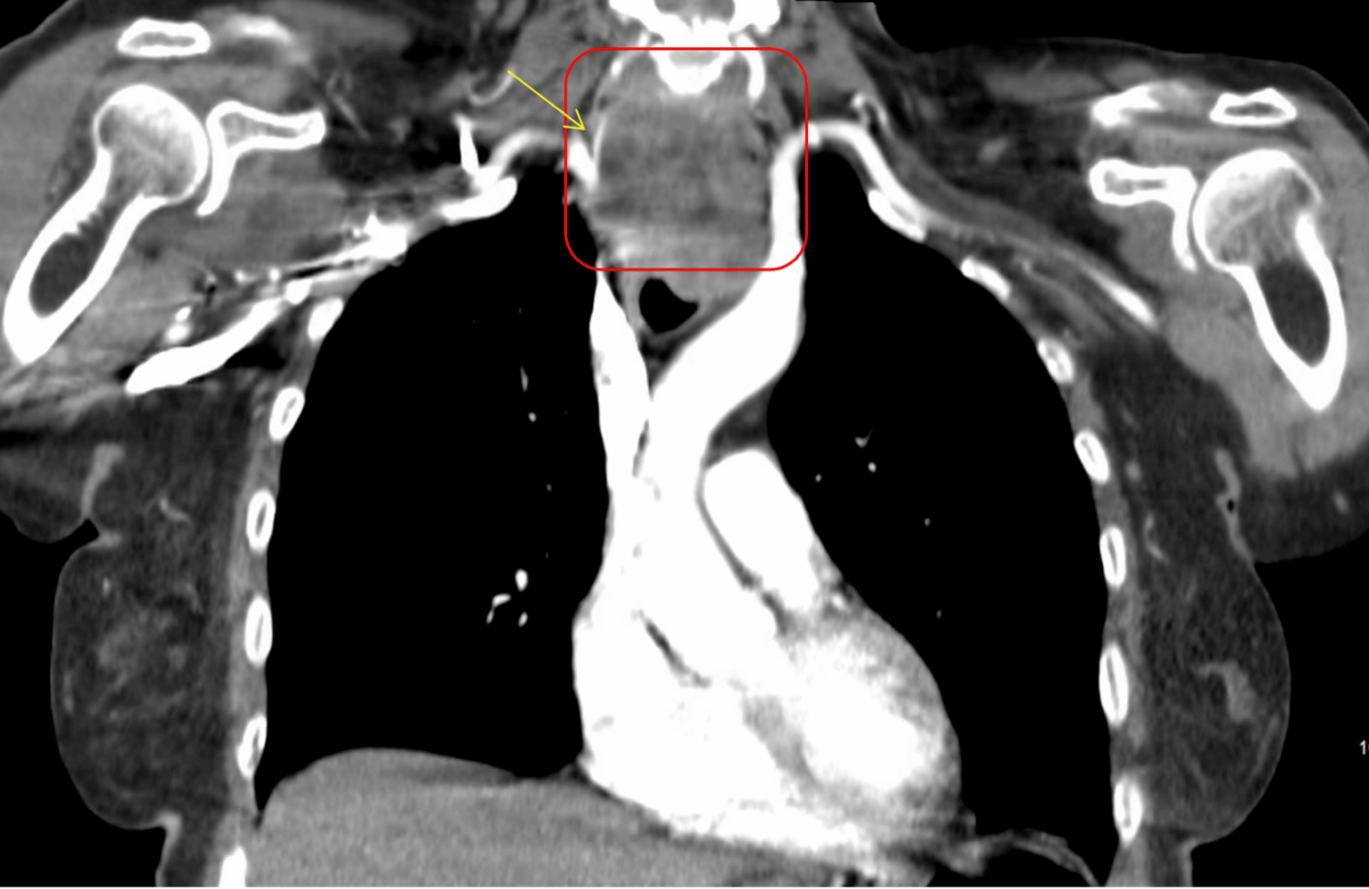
The CTA pulmonary (coronal view) shows a large right paratracheal mass (red box). The mass demonstrates a superior lateral extension into the right supraclavicular fossa, with near complete encasement of the proximal right common carotid artery (yellow arrow). CTA: computed tomographic angiography
